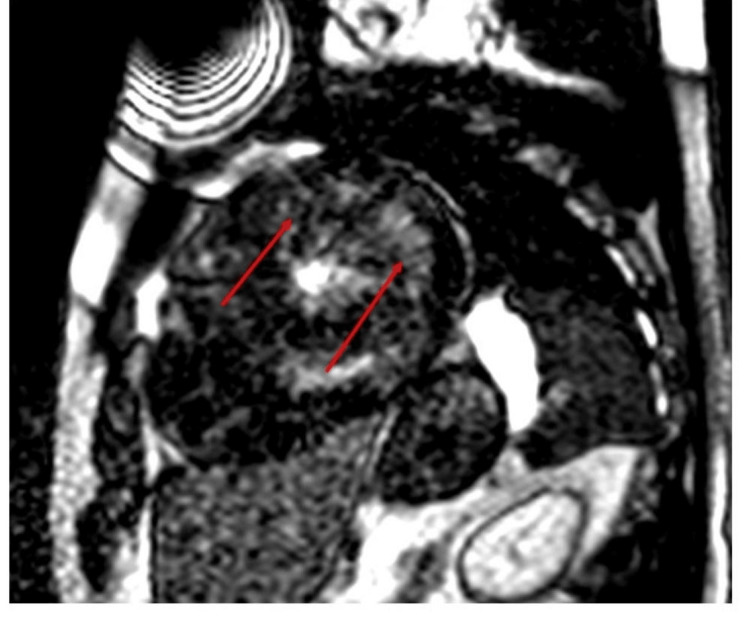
Cardiac MRI short axis showing late gadolinium enhancement (LGE) at apex (red arrows).
